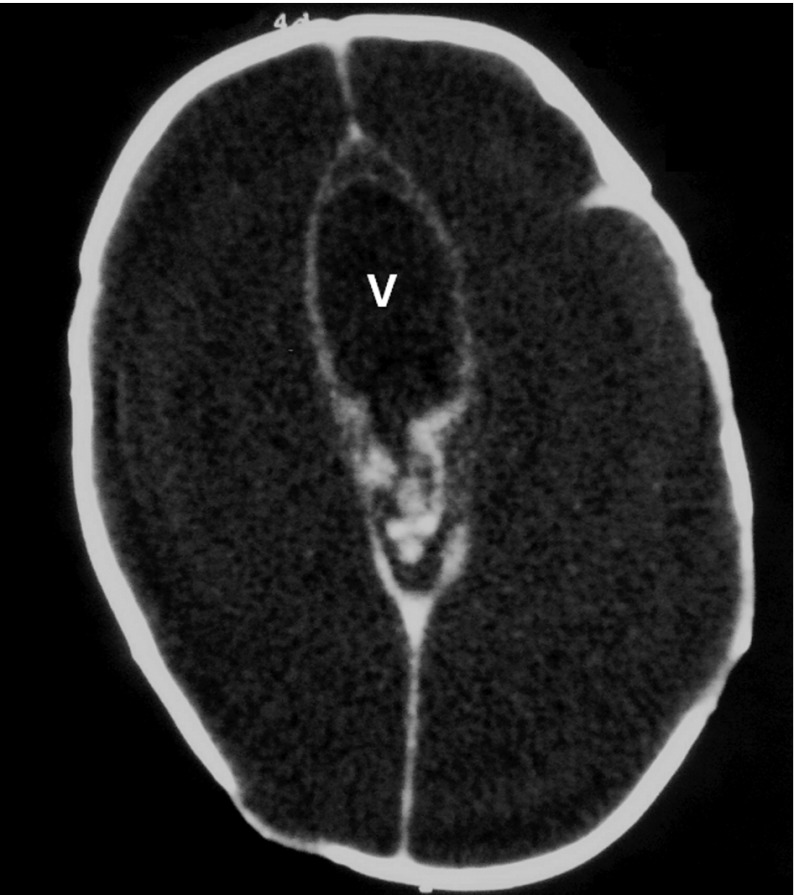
CT image of 2 days old newborns brain. Dilated third ventricle (V) with absent thalami and basal ganglia and cerebral cortex.
